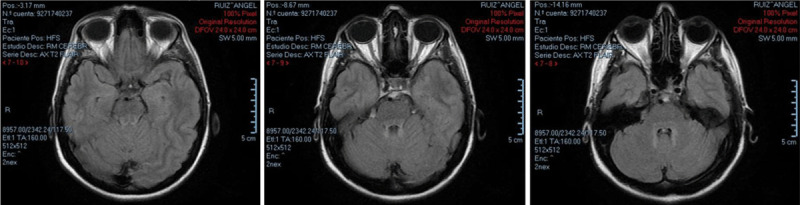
Case 2 brain MRI with bilateral T2/FLAIR hyperintense signal located in the superior cerebellar peduncle, around the IV ventricle, and dentate nuclei.
